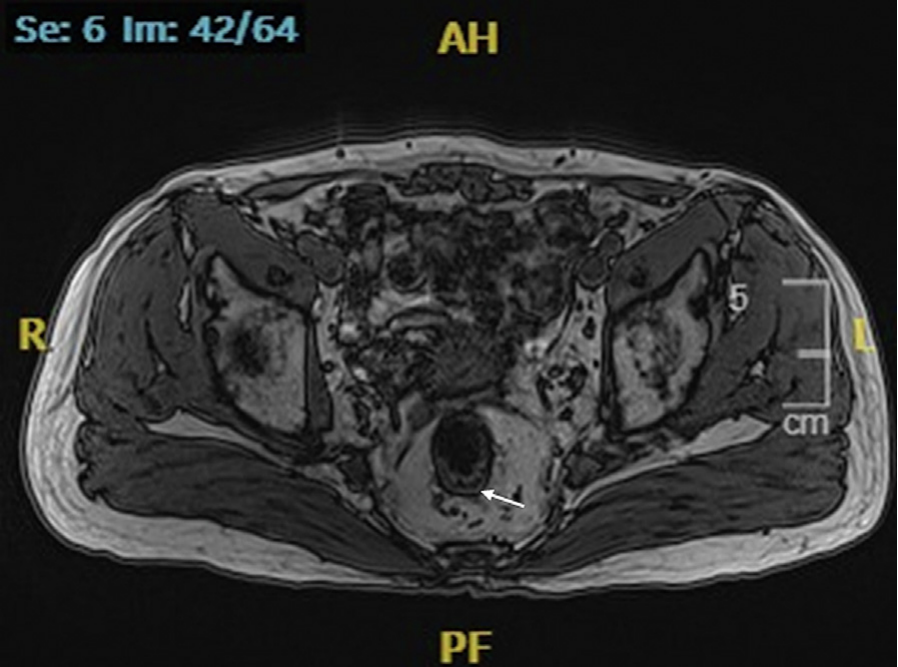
Magnetic resonance imaging showing a 3.5-cm-long circular growth in the rectum 8.5 cm from the anus (*white arrow*).
